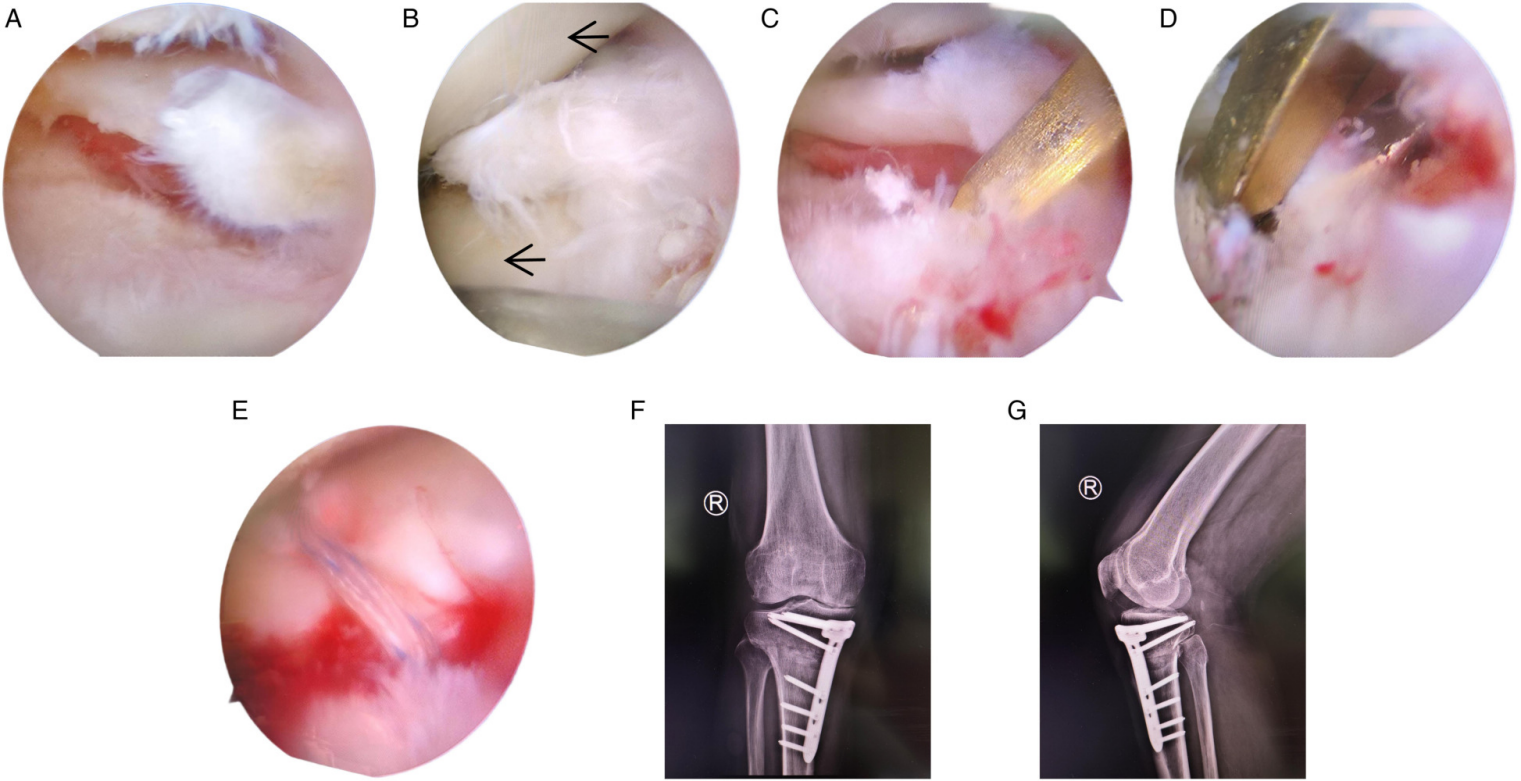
Intraoperative arthroscopic findings and immediate postoperative radiographs. (A) The meniscus was torn at the attachment point of the posterior root; (B) cartilage in the lateral compartment of the knee is well preserved (marked by red arrows); (C,D) two bone tunnels were made at the meniscus attachment using a locator; (E) the meniscus sewing thread pulls out of the bone tunnel; (F,G) postoperative radiographs, showing that the osteotomy was fixed with a TomoFix plate.
